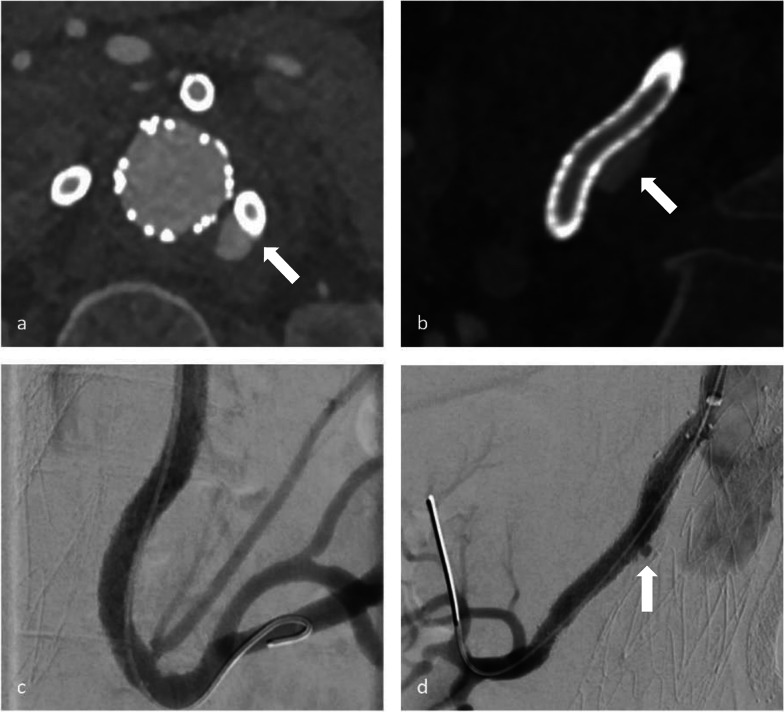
Endoleak adjacent to the left renal BSG in CTA (a, b). While the endoleak could not be confirmed in angiography (c), a previously undetected endoleak of the right renal BSG was found (d). Both BSGs were successfully religned. BSG = bridging stentgraft, CT = computed tomography, CTA = computed tomography angiography
